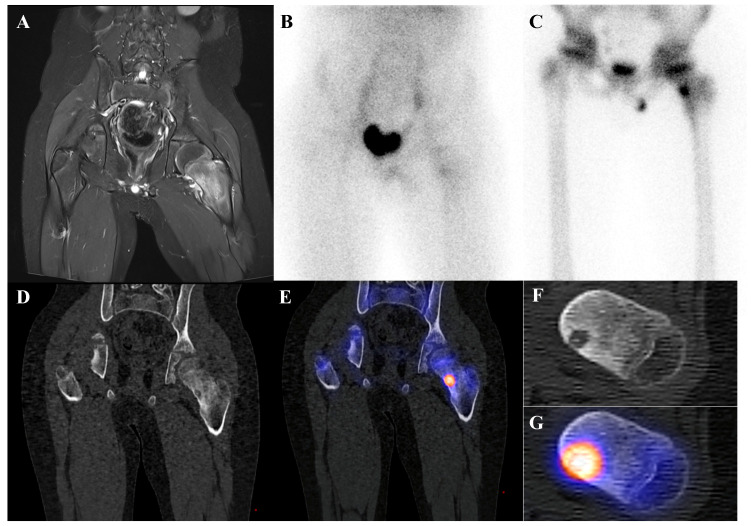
An 11-year-old male with osteoid osteoma of the left femoral neck. (A) T2-weighted fat-suppressed magnetic resonance imaging shows a lesion with high signal intensity and a sclerotic rim in the medial cortex, accompanied by perilesional edema (“half-moon sign”). (B,C) Bone scintigraphy demonstrates increased uptake in the left femoral neck on both blood pool and delayed phases. (D–G) Bone single-photon emission tomography/computed tomography reveals an osteolytic lesion with a central nidus and intense uptake.
